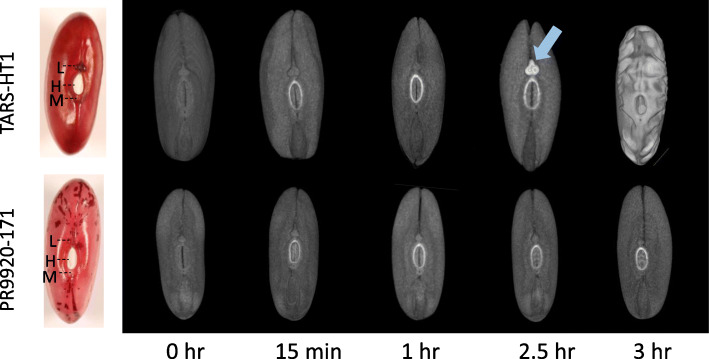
Tracking seed water uptake in PR9920–171 and TARS-HT1 using iodine contrast and CT-scan imaging. Within one hour of soaking in iodine solution, a bright signal around the hilum was detected in both genotypes. At 2.5 h, a change was detected on the lens (arrow) of TARS-HT1 seeds which indicates the site of water entry in the seed coat. After 3 h of soaking, the water penetrated the whole seed coat of TARS-HT1 while PR9920–171 seeds remained unimbibed. The seeds of both genotypes were represented on the left as a reference. Several structures are distinguishable in legume seeds. H = hilum, L = Lens and M = micropyle
